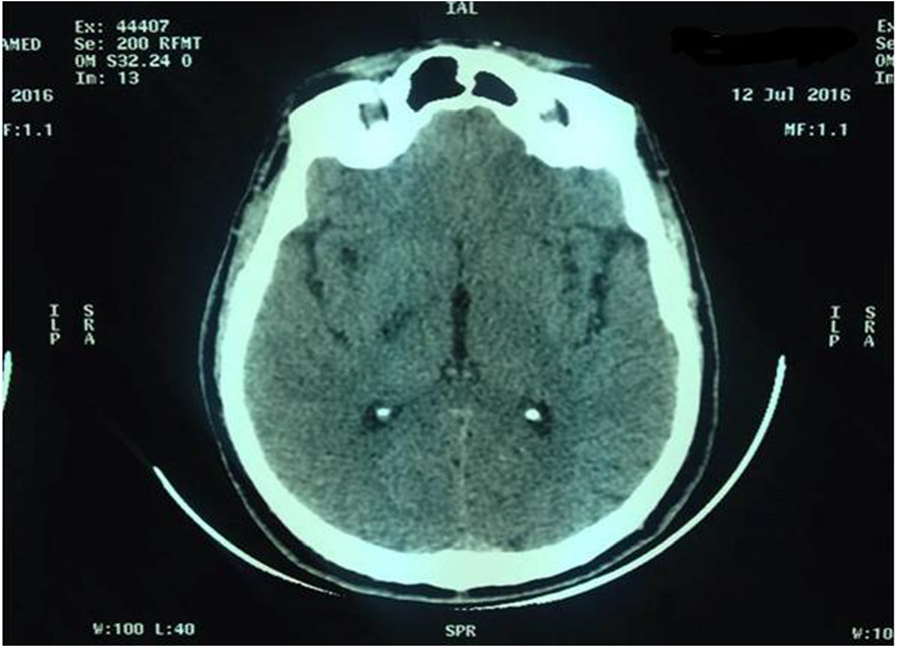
Cerebral computed tomography showing a right internal capsule ischemic stroke
